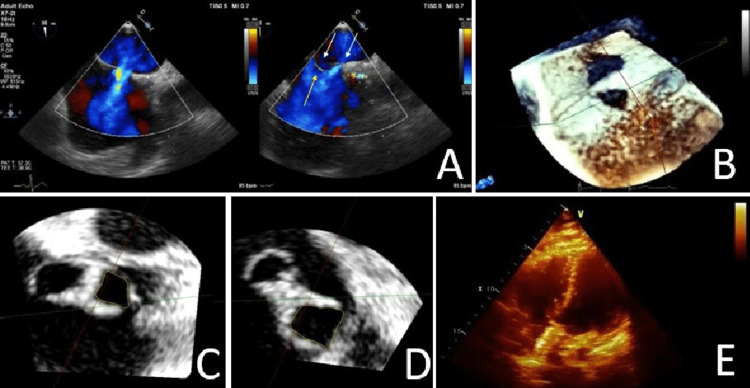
Echocardiography images 1A. Two-dimensional TTE with Doppler demonstrated two interatrial septal defects (white arrows) and a septal aneurysm (yellow arrow). 1B. Three-dimensional TEE demonstrated two interatrial septal defects. 1C. Three-dimensional TEE demonstrated one ASD measured at 0.44 cm^2^. 1D. Three-dimensional TEE demonstrated one ASD measured at 0.74 cm^2^. 1E. Post-procedural TTE demonstrated stable positioned occluder and septum.
